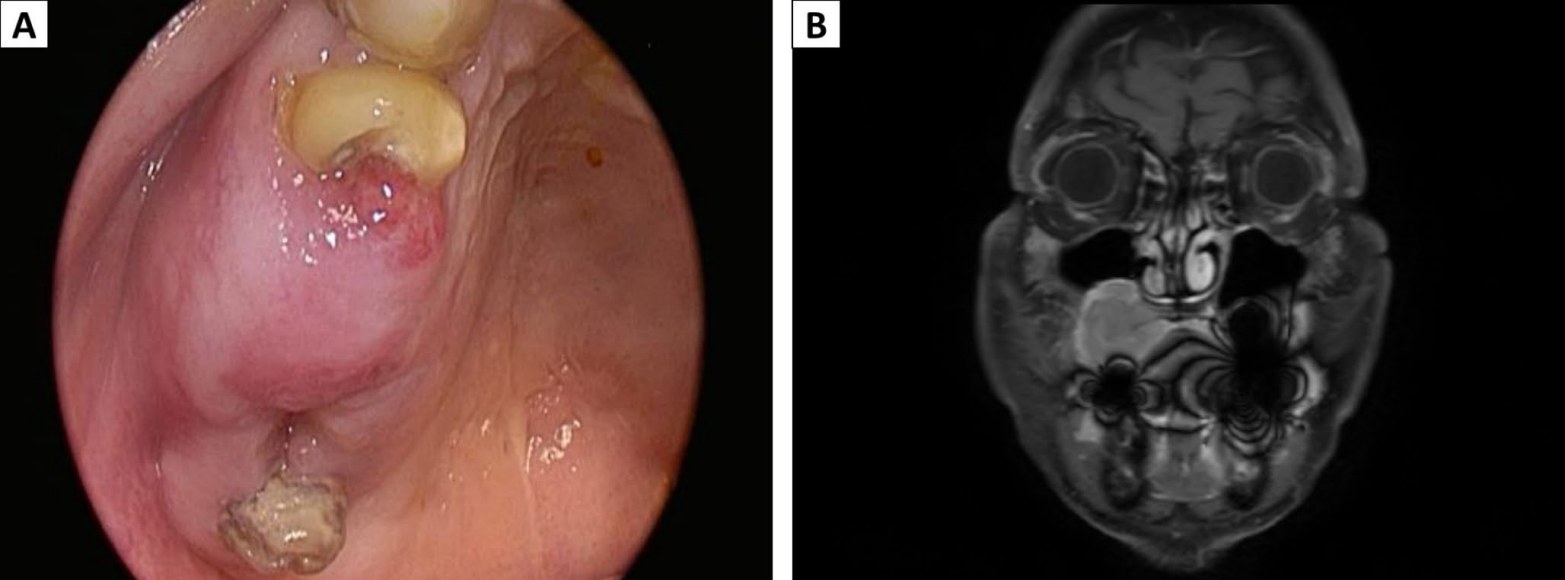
Clinical presentation. (A) Intraoral view showing redness and swelling of the gingival mass with ulceration in the posterior portion. (B) Coronal magnetic resonance T1-weighted image with contrast enhancement showed a right maxillary mass
